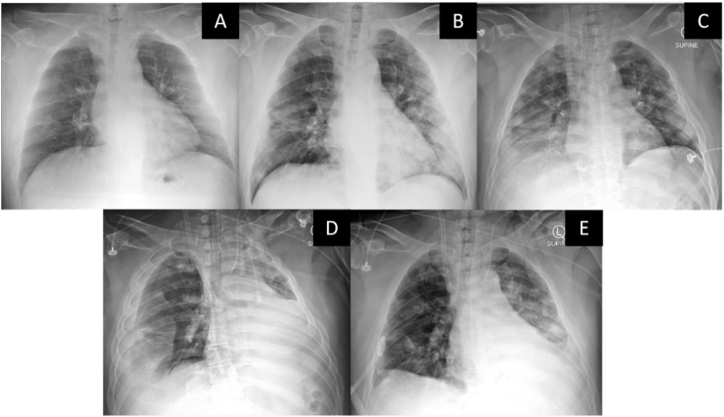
Serial portable chest radiographs of the patient. (A) Hospital day 1 (day 8 after the symptom onset). (B) Hospital day 8. (C) Hospital day 14 (ECMO day 1). (D) Hospital day 23 (ECMO day 10) – right hemothorax with left shift of mediastinum were noted and (E) after insertion of a percutaneous drainage tube to the right pleural space.
